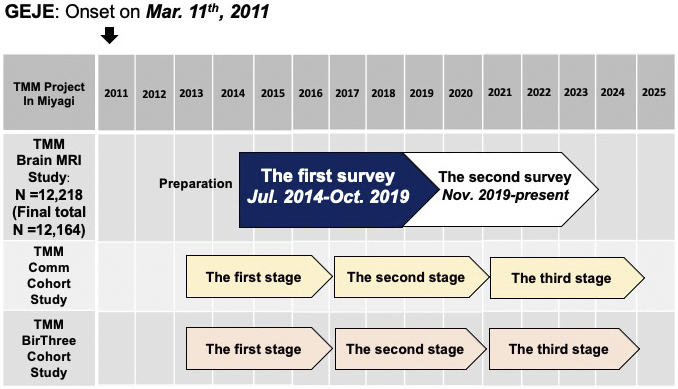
Timeline of the TMM Brain MRI Study and its relation to the TMM CommCohort Study and TMM BirThree Cohort Study. The timeline of the TMM Brain MRI Study is presented in comparison with the timeline of the TMM CommCohort Study and the TMM BirThree Cohort Study. The first (baseline) survey of the TMM Brain MRI Study was conducted from July 2014 to Oct. 2019. It was conducted until late October 2019. The second (first follow-up) study began on the last business day of October 2019. Please note that the start and end dates for the TMM Brain MRI Study are later than the start and end dates for the two cohort studies.
